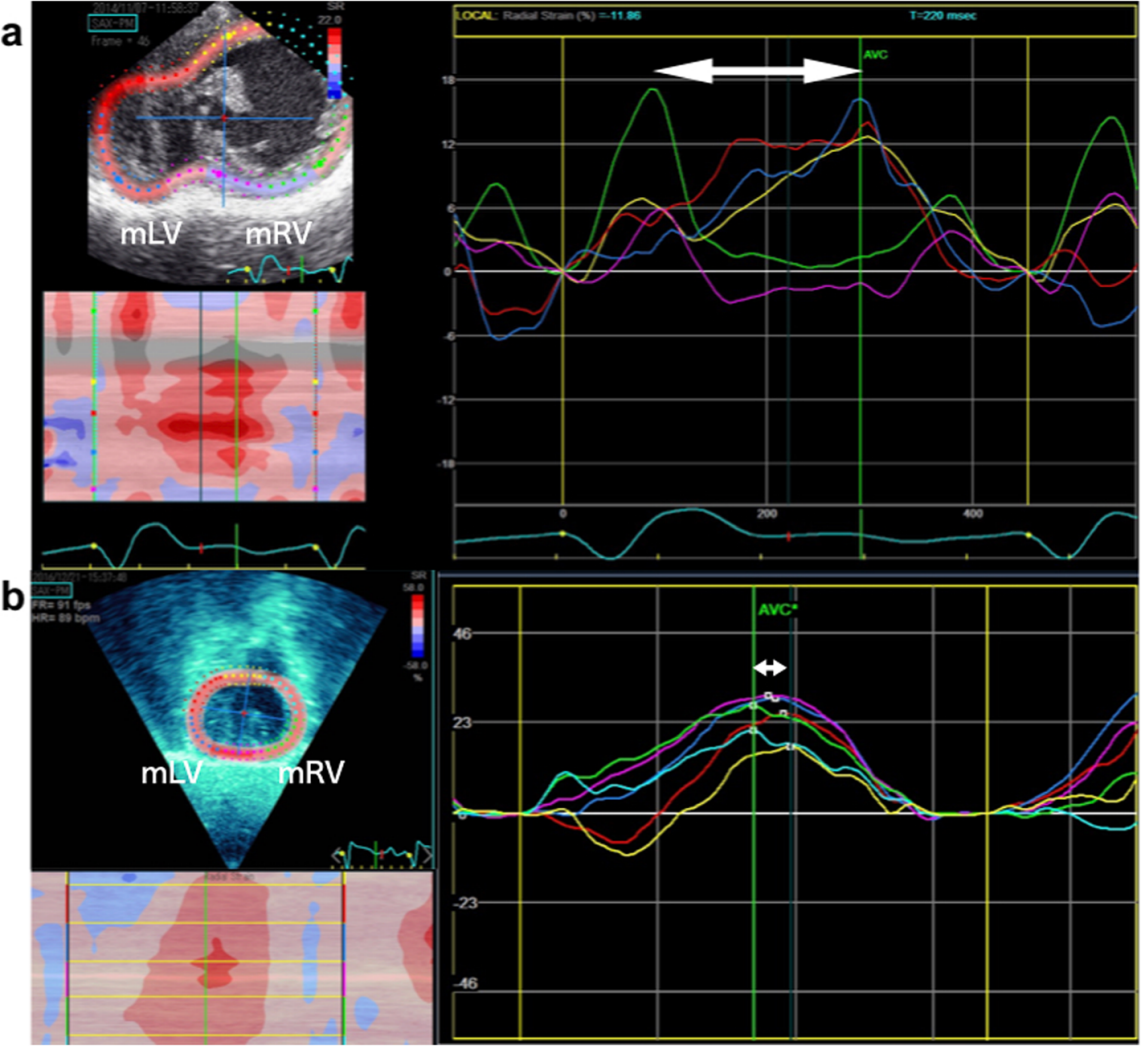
a Speckle tracking echocardiography reveals that the difference in time to peak radial strain between both ventricles is 210 ms before CRT (white arrow). b Speckle tracking echocardiography after CRT shows good interventricular synchrony between both ventricles. The peak radial strain between both ventricles is very short (white arrow). CRT, cardiac resynchronization therapy; mLV, morphological left ventricle; mRV, morphological right ventricle
